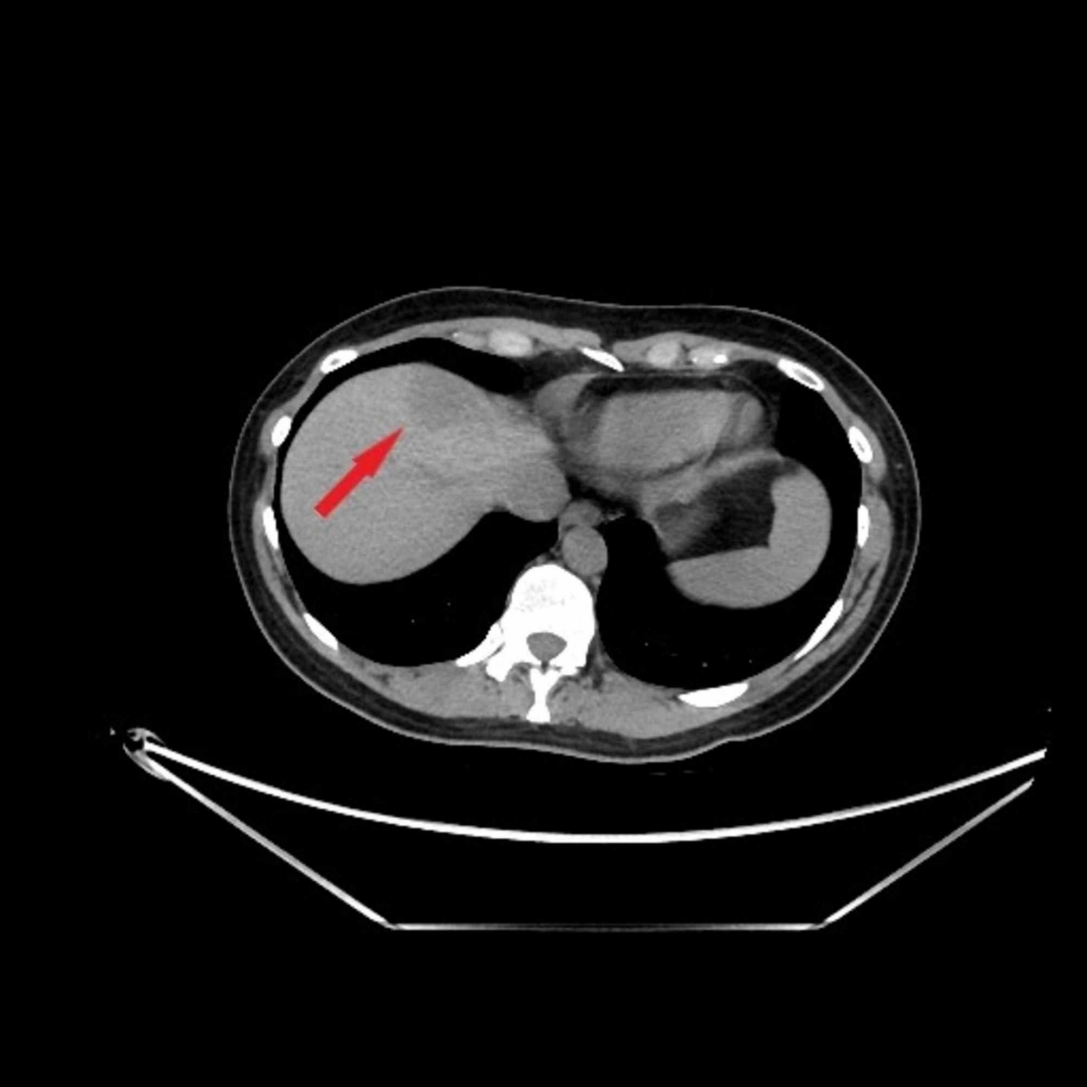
CT scan Hypo-attenuating lesion in the dome of the right hepatic lobe measuring approximately 2.6 x 3.2 x 2.6 cm.
